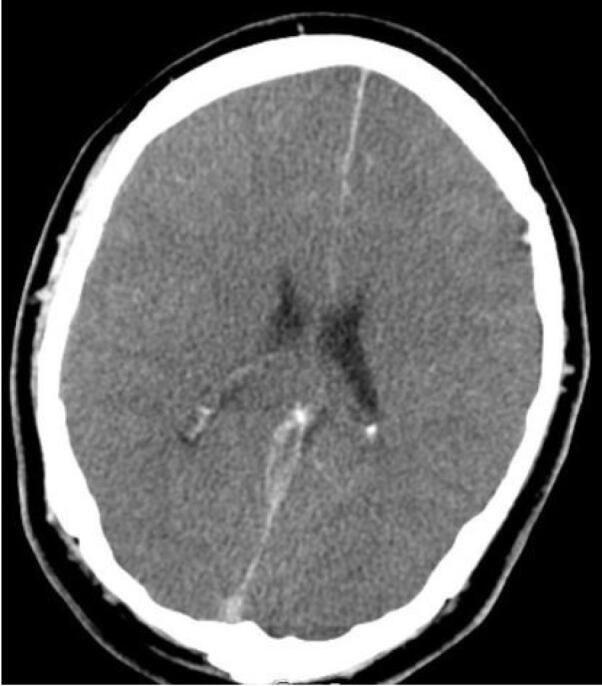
Table 3 T3: Distribution of Brain CT Findings in Methanol Poisoning Patients
